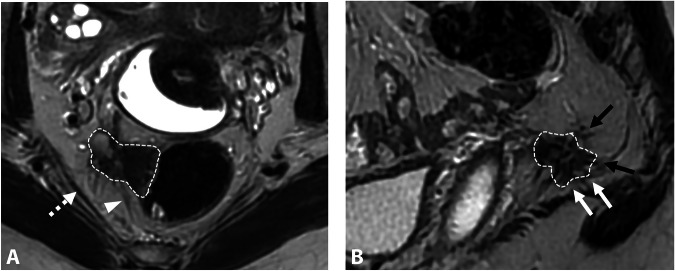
DE in a 29-year-old woman with dysmenorrhea, deep dyspareunia, and dyschezia. A Axial and (B) sagittal T2W MR images show a right subperitoneal infiltrative lesion (dotted lines) involving the anterolateral rectal wall and extending to the mesorectal fascia (A, white arrowhead) and beyond into the right posterolateral parametrium. The infiltration of the inferior hypogastric plexus shows spiculated margins (B, black arrows) but no extension to the sacral roots or the pelvic wall (A, dashed arrow). Note the safety fat line between the lesion and the iliococcygeus muscle of the levator ani muscle (B, white arrows). Laparoscopic surgery confirms the involvement of the inferior hypogastric plexus and a cleavage plane with the levator ani muscle and sacral roots
